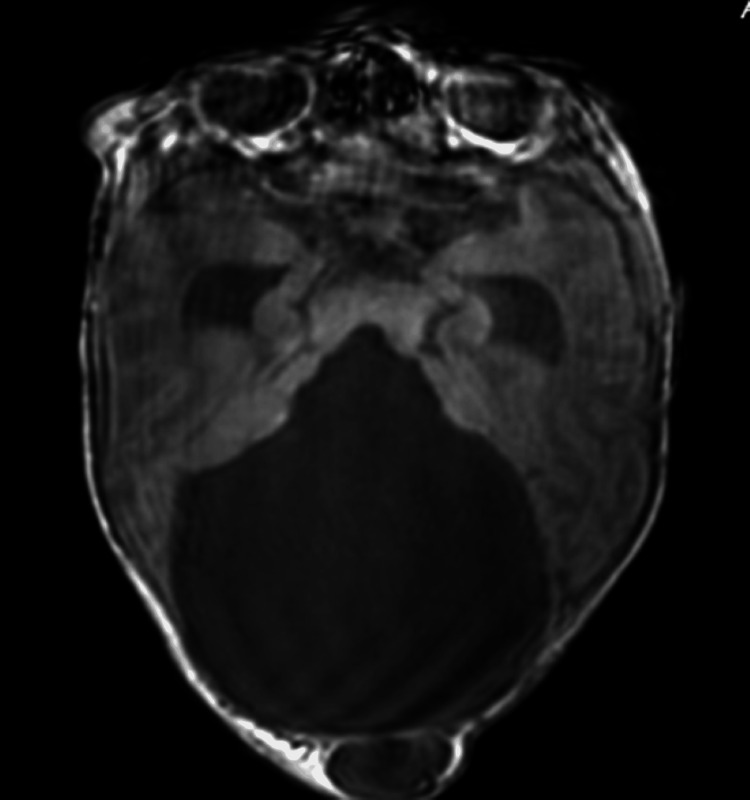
MRI axial image demonstrating the presence of a severe Dandy-Walker malformation and the occipital cephalocele
